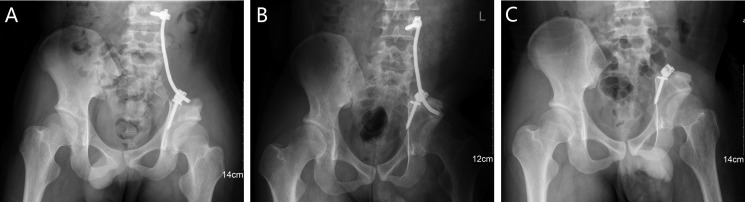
A 20-year-old male (case 25) with diagnosis of pelvis osteosarcoma affecting zone I (A) AP radiograph at two weeks post-operatively. (B) AP radiograph at 30.5 months post-operatively, showing failure of the implant and with functional impairment. (C) AP radiograph at one week after removal of the implant.
